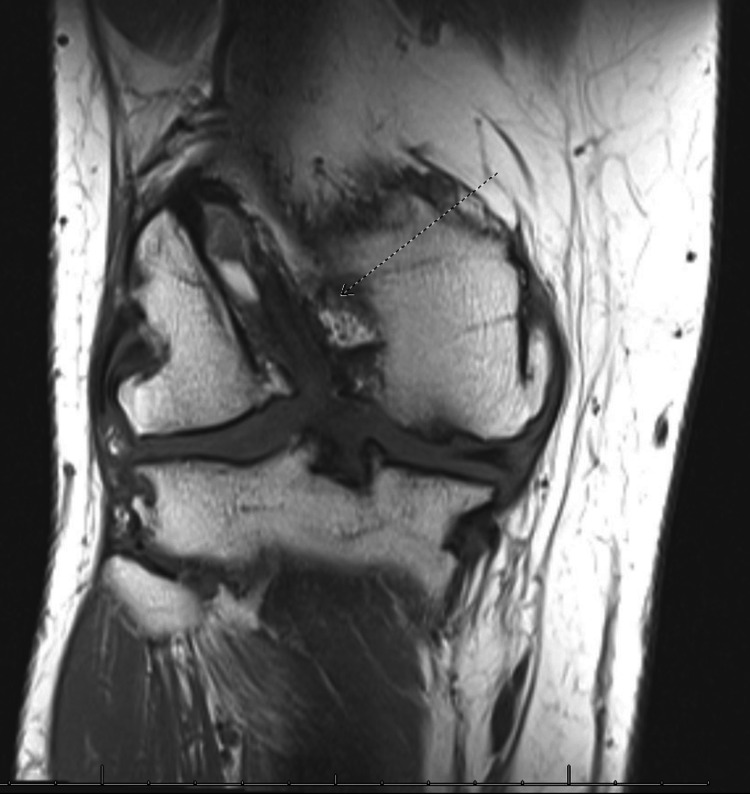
MRI right knee, anteroposterior view showing the previous femoral tunnel (arrow).
